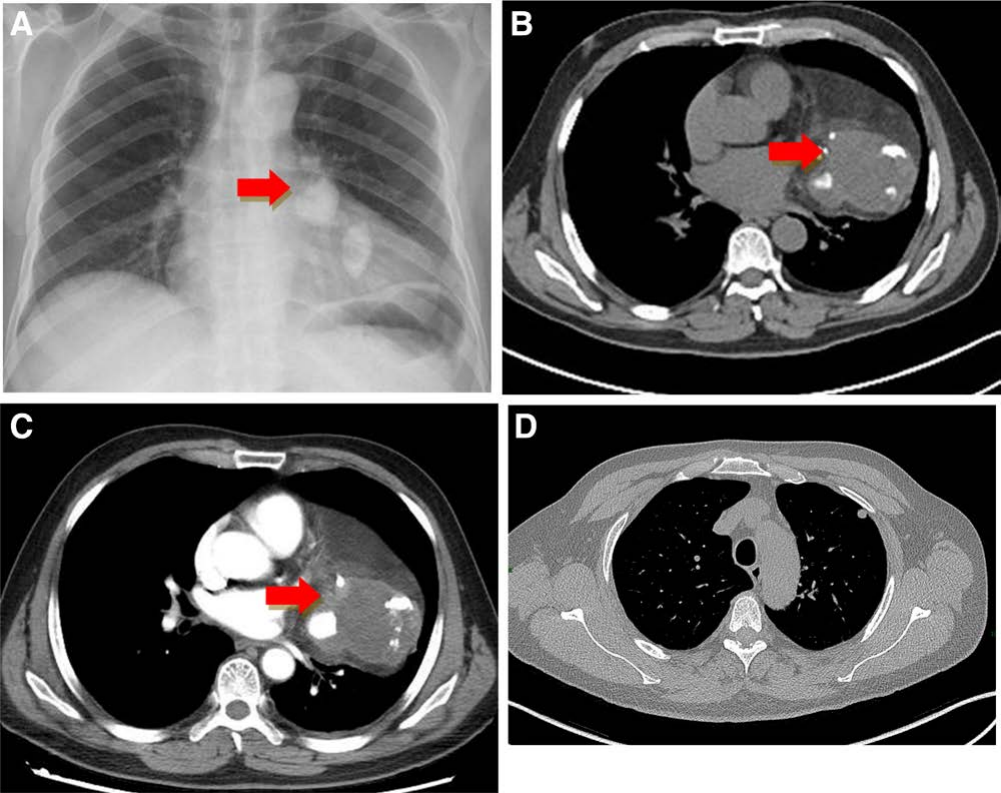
Chest X ray and CT. (A) Chest radiograph showing a high-density shadow. Regular (B) and contrast-enhanced (C) views of a chest CT scan demonstrating a heterogeneous mass (13.9 cm × 8.4 cm × 8.1 cm) in the anterior mediastinum, containing soft tissue (asterisks), fat, peripheral calcification, and low-attenuation areas of probable necrosis. A capsule is present; it is incomplete and insufficient to contain the tumor. (D) A solid nodule of the left upper lobe. CT = computed tomography.
